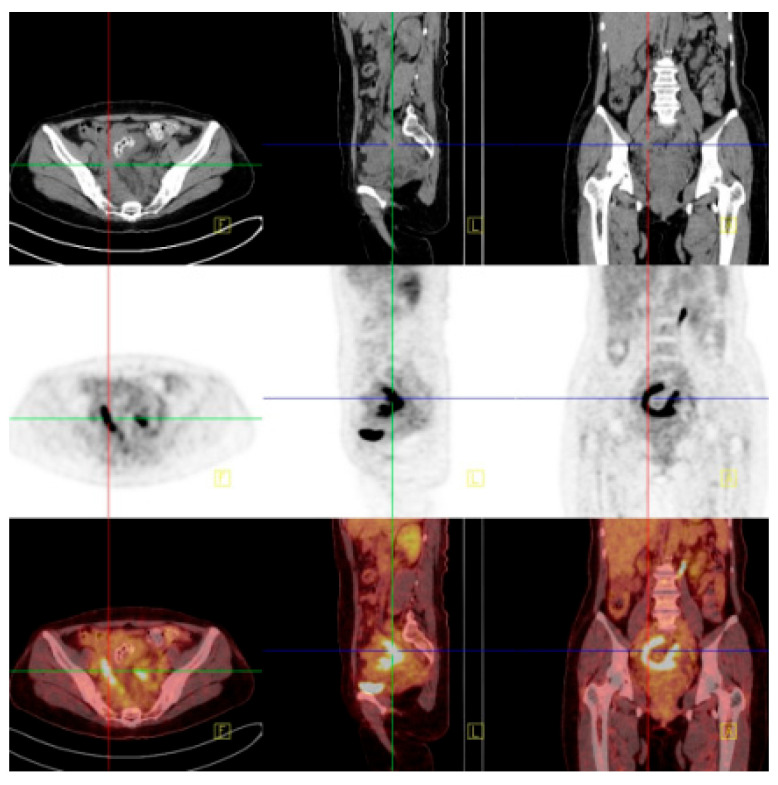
Positron emission tomography–computed tomography (PET-CT) reveals an irregular high-density mass in the pelvic mesentery, forming a ‘C’ shape enveloping the sigmoid colon from the right, with unclear demarcation from the sigmoid colon and markedly increased FDG uptake. FDG can be used as an important indicator to distinguish benign and malignant lesions by reflecting the level of glucose metabolism in the lesion, but it does not have absolute specificity. Tuberculosis and inflammatory lesions can also show increased FDG. There may be chronic granulation tissue hyperplasia near actinomycete abscesses or sinuses, and the infiltration of various inflammatory cells, tissue cells, and fibroblasts, resulting in high uptake of 18F-FDG.
